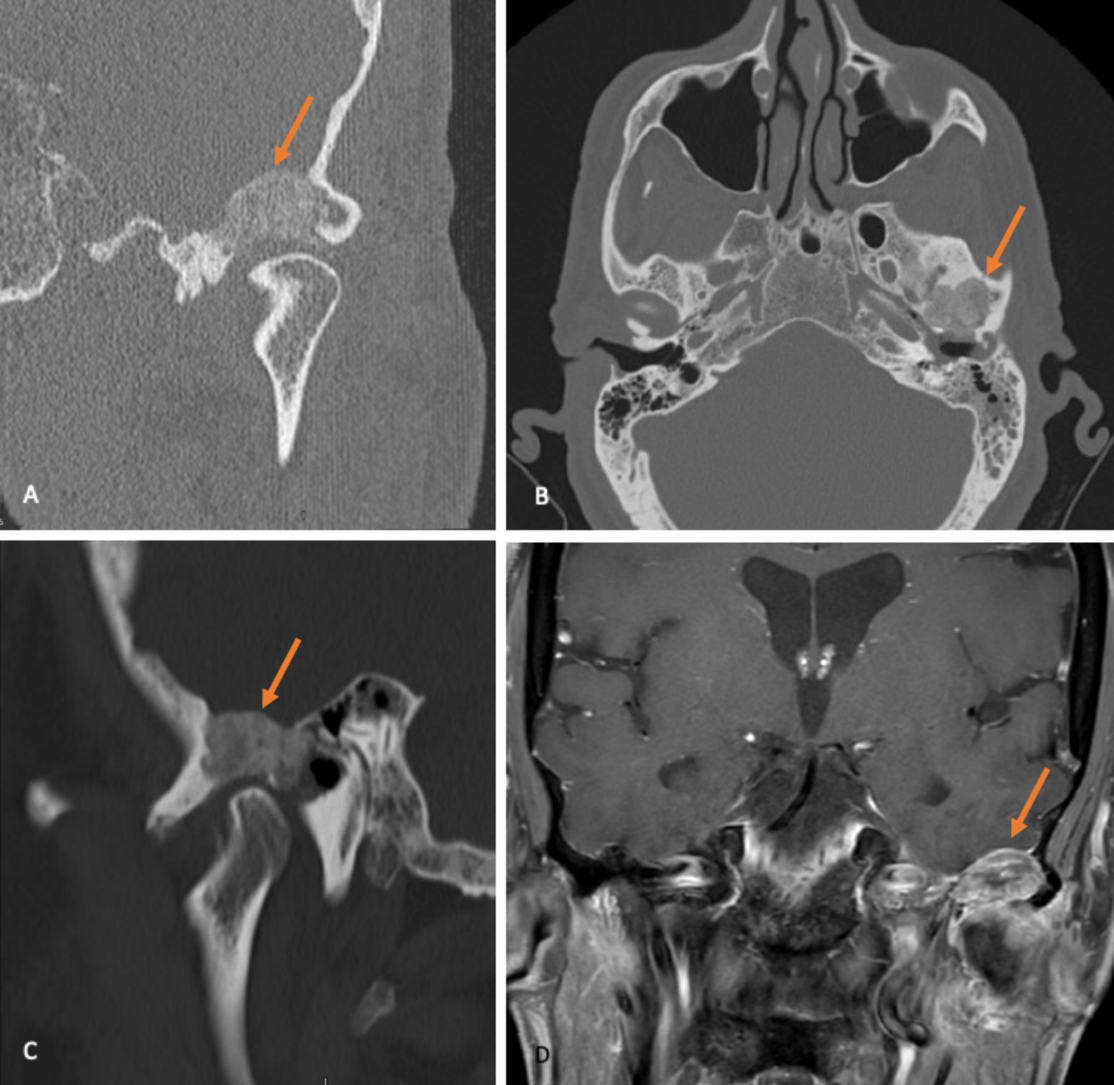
Preoperative imaging left temporal area. Non-contrast head CT: (A) coronal view of a left para-articular temporal lesion and (B) axial view showing erosion of the anterior external auditory canal wall and opacification of the mastoid air cells; (C) sagittal view and (D) sagittal T1-weighted MRI with gadolinium showing lesion extension towards the middle cranial fossa apparently without signs of dural or cerebral involvement.
